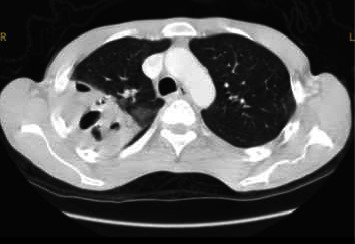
Chest computed tomography: segmental necrotizing pneumonia with cavitation of the right upper lobe.
